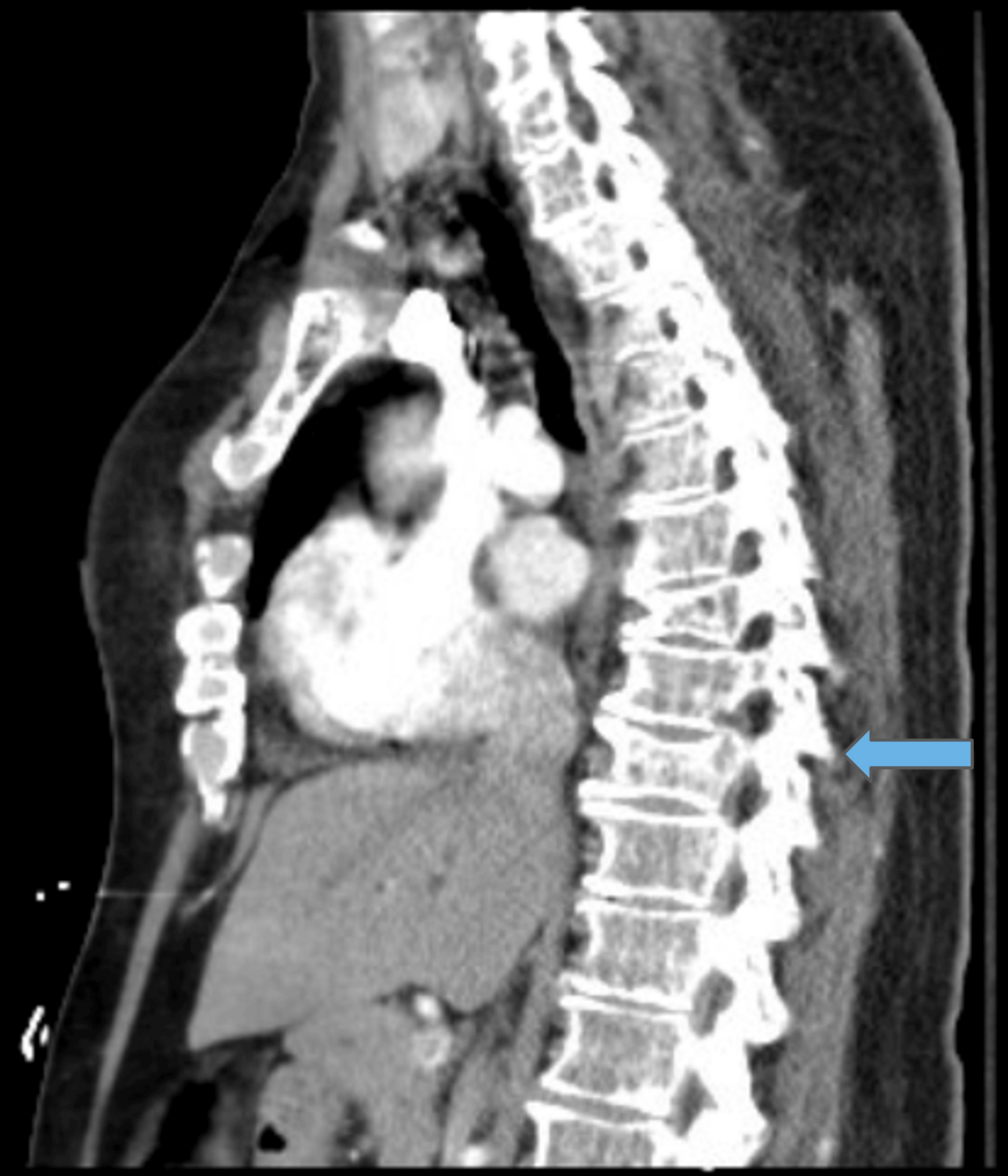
CT chest (sagittal view) demonstrating moderate compression deformities in the thoracic spine (blue arrow).
